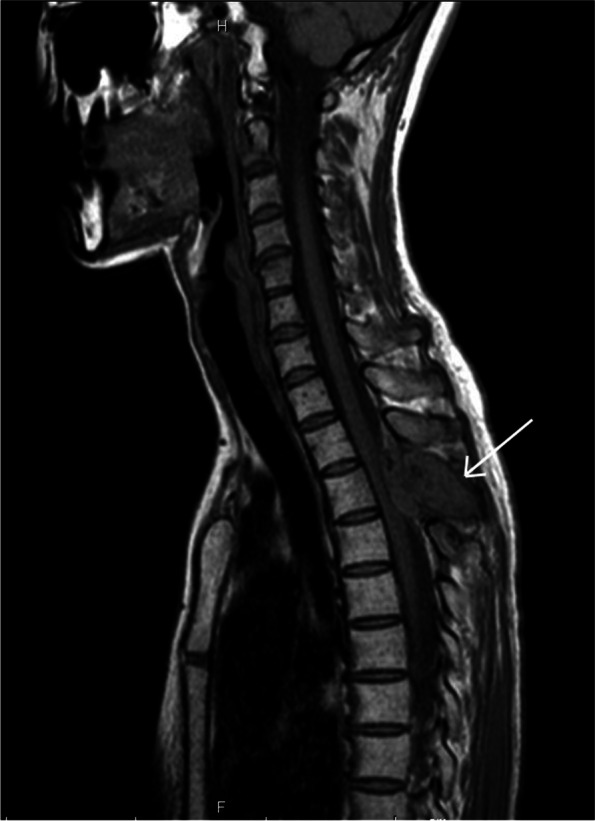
Preoperative sagittal T1-weighted magnetic resonance imaging revealed a mass in the posterior portion of the third thoracic vertebra
